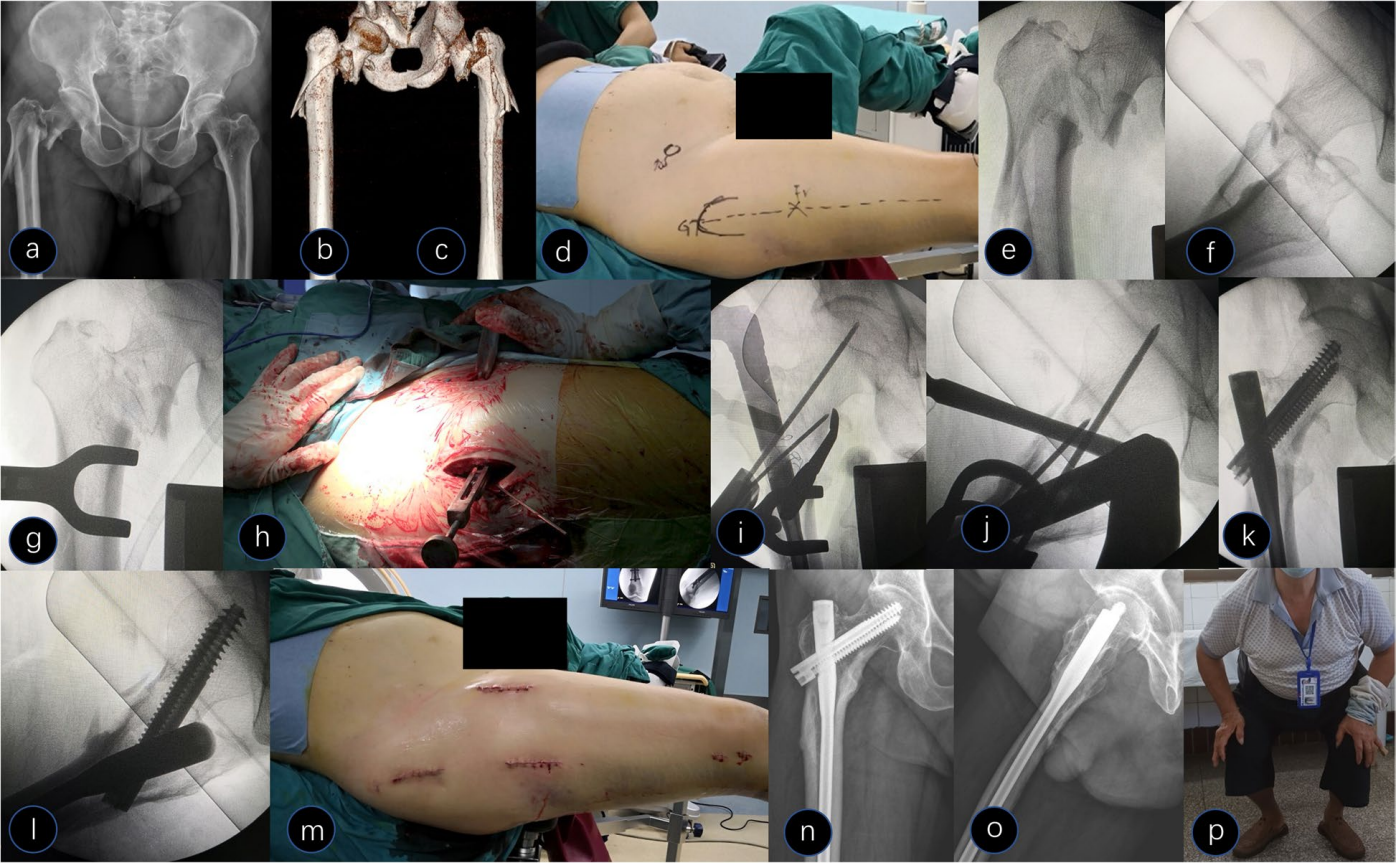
A 68-year-old male presented with a Type V fracture. a-c Preoperative X-ray and computed tomography 3D reconstruction showed medial-lateral displacement of the subtrochanteric region and medial-lateral displacement of the intertrochanteric region. d Picture of the surgical posture. e and f Closed reduction with traction under fluoroscopy. g A Lowman clamp was used for the subtrochanteric fracture, and the intertrochanteric fracture was irreducible (iliopsoas muscle embedded into the lesser trochanter). h Surgical picture of the maintained reduction in both regions. i and j Intraoperative X-ray after both regions were reduced. k and l Intraoperative X-ray after reduction and fixation. m Postoperative picture of the incision. n and o Postoperative X-ray at 48 months. *p* Functional picture at follow-up
